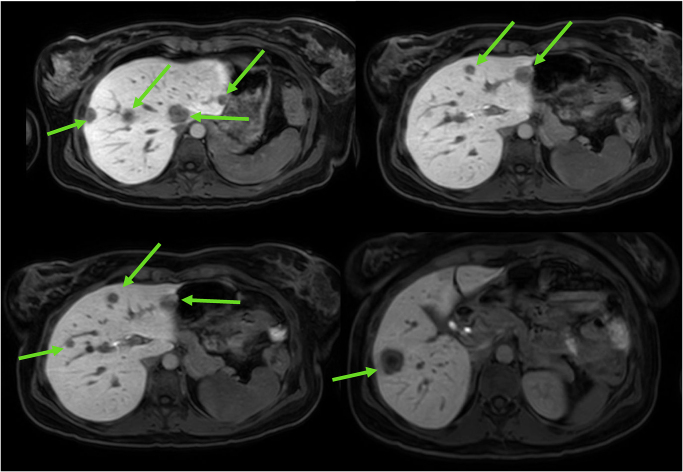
Primovist-enhanced liver MRI scan at diagnosis. Green arrows show the distribution of liver metastases (Not all liver metastases shown).
